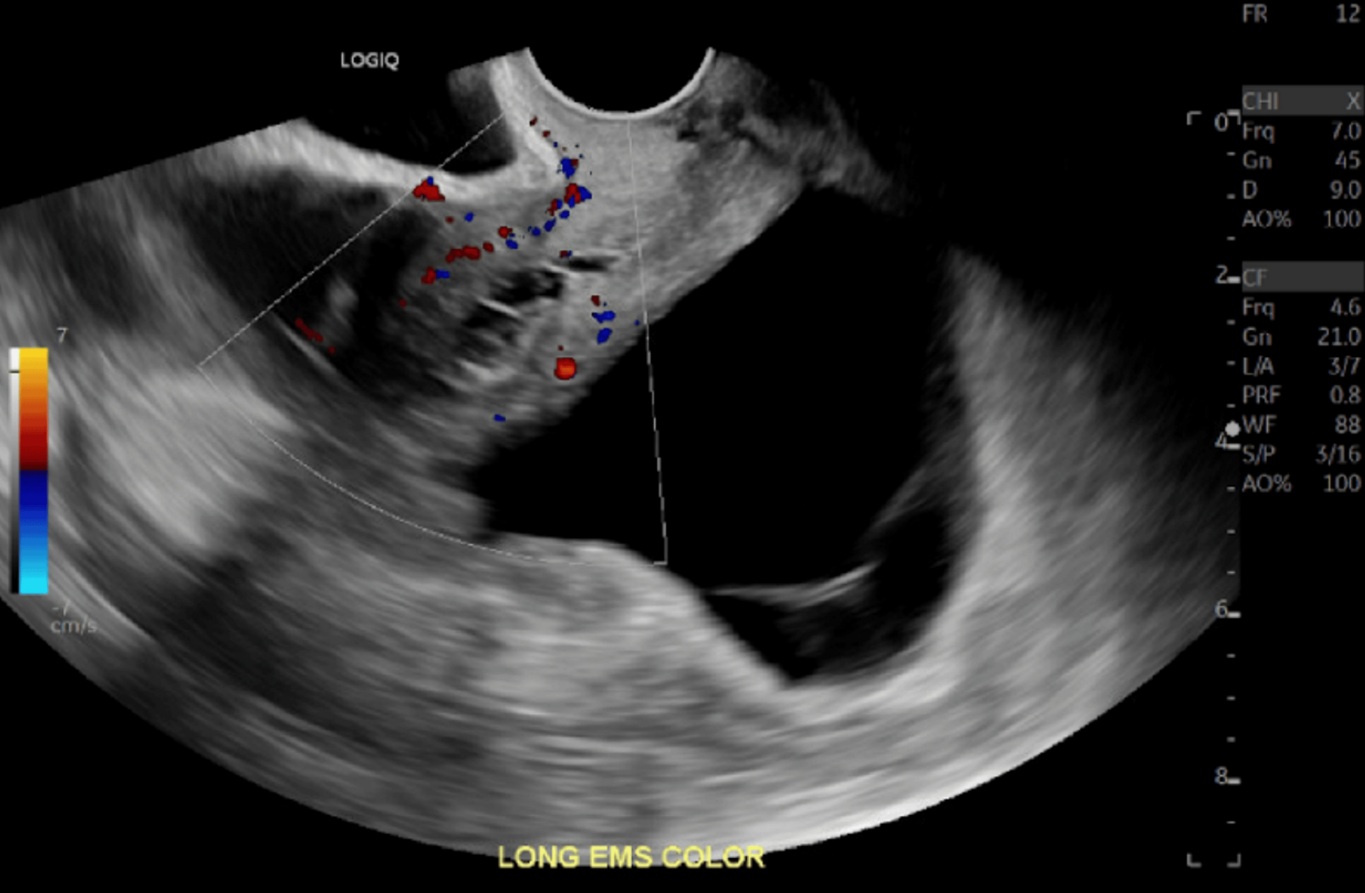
Pelvic ultrasound of the uterus with color Doppler showing a thickened endometrial stripe
